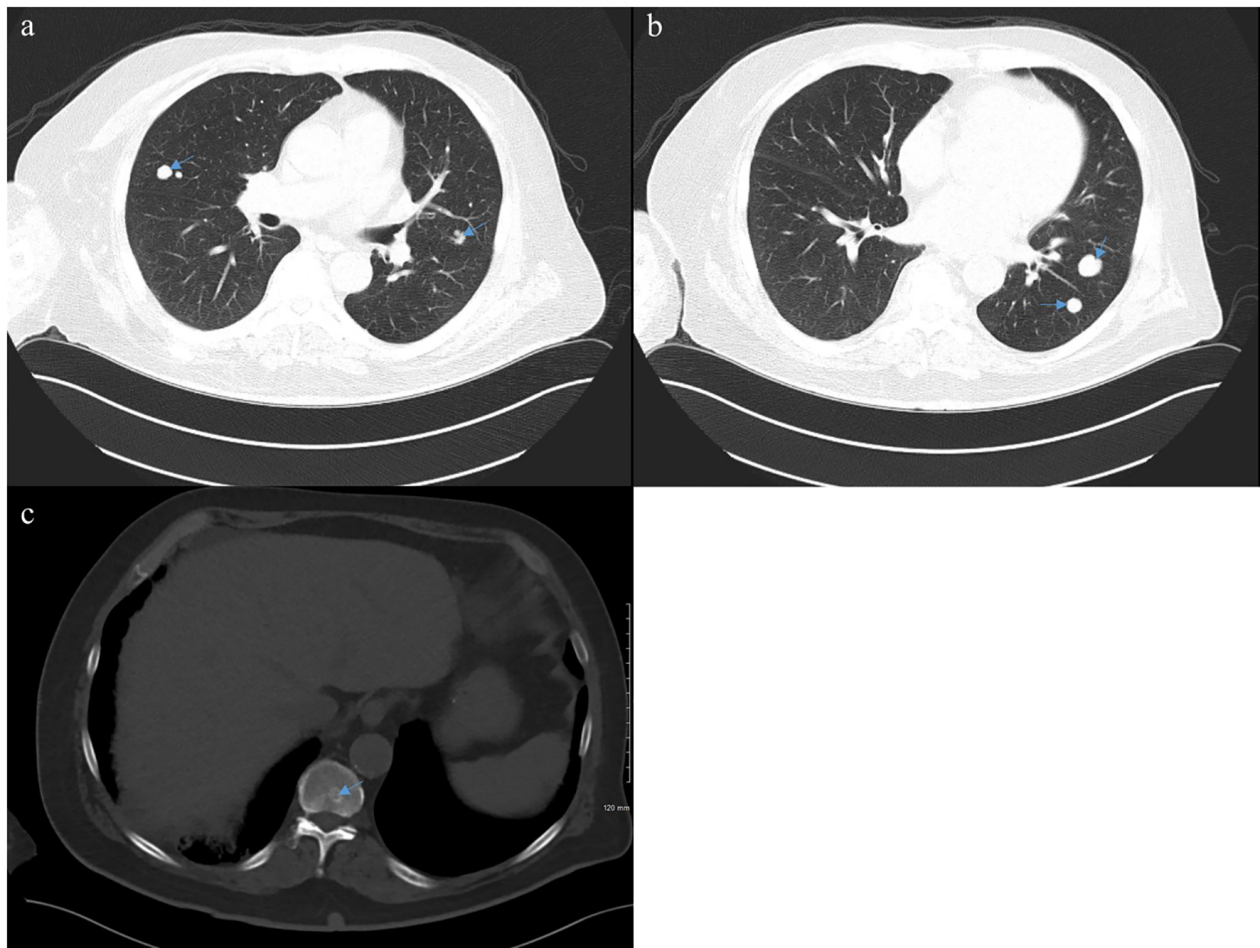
Computed tomography (CT) of the chest, abdomen, and pelvis from April 2021 demonstrating bilateral pulmonary nodules (A, B) and an L2 lesion (C) consistent with metastatic disease.
